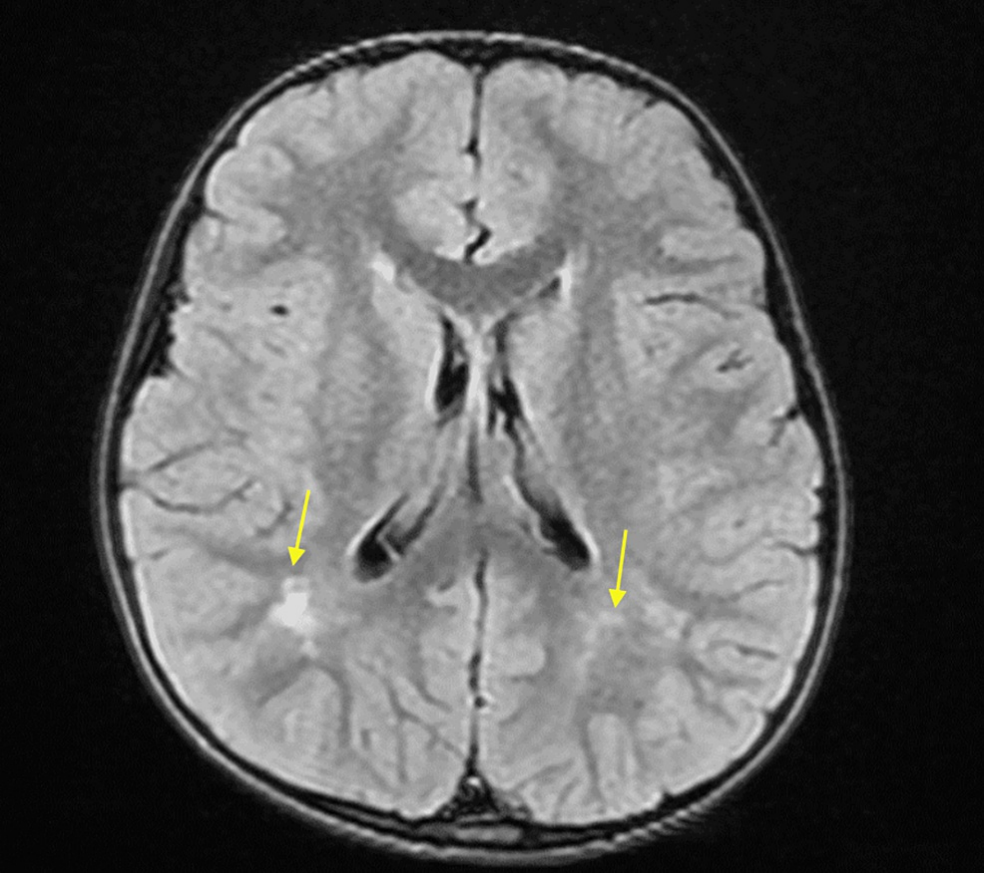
Brain MRI of the patient's five-year-old sister High signals on the bilateral parietal lobe.
